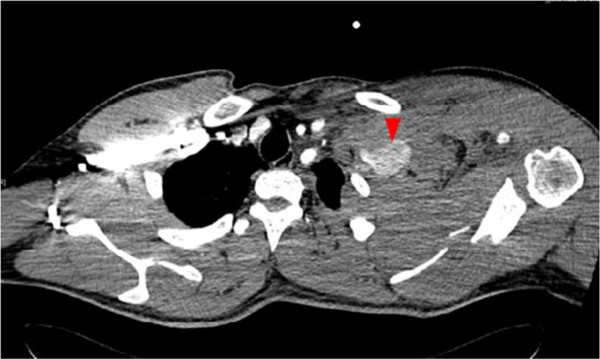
Polytrauma CT scan. Extravasation of contrast agent in left subclavian artery indicating rupture.
